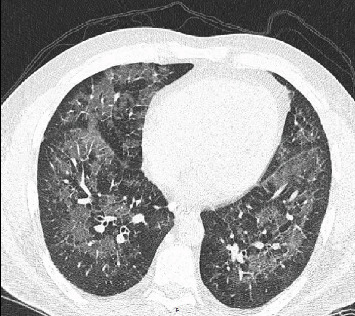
Case 1 chest CT scan on presentation (axial view).
